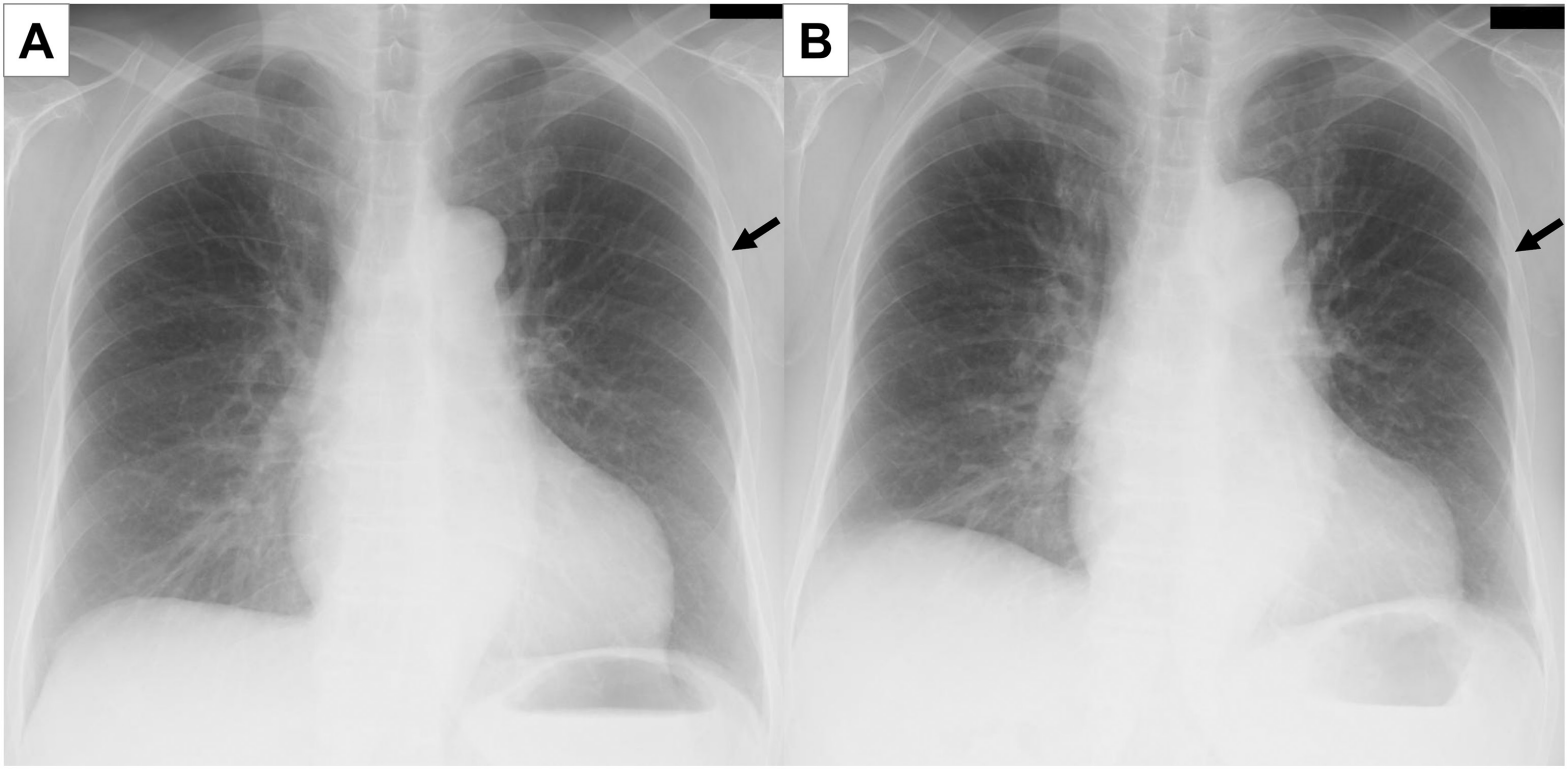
Serial chest radiographs showing interval growth of a left upper‐lung ground‐glass nodule. (A) Seven years prior to presentation, a ground‐glass nodule is observed in the lateral left upper lung field (black arrow). (B) At presentation, the ground‐glass nodule has enlarged (black arrow).
